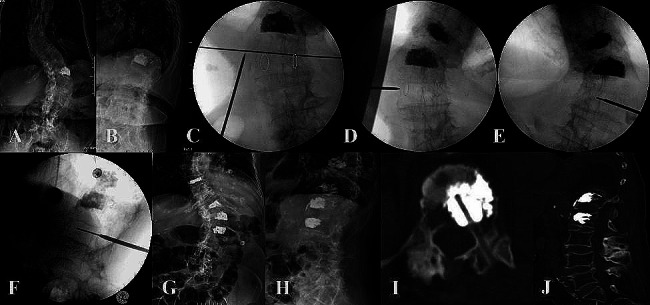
A and B, the X-ray of PA and lateral view at pre-operation; C, the intraoperative PA image revealed asymmetrical pedicle shadows; D, C-arm position is rotated and both pedicles are nearly symmetrical; E and F, the guide wire was at or cross the midline in PA view (E) and reached the anterior one-fourth of the vertebral body at the lateral view (F); G and H, the PA (G) and lateral (H) X-ray after surgery; I, the axial CT image revealed adequate cement distribution and the puncture track; J, the sagittal CT reconstruction image revealed the vertebral height and sufficient cement distribution
